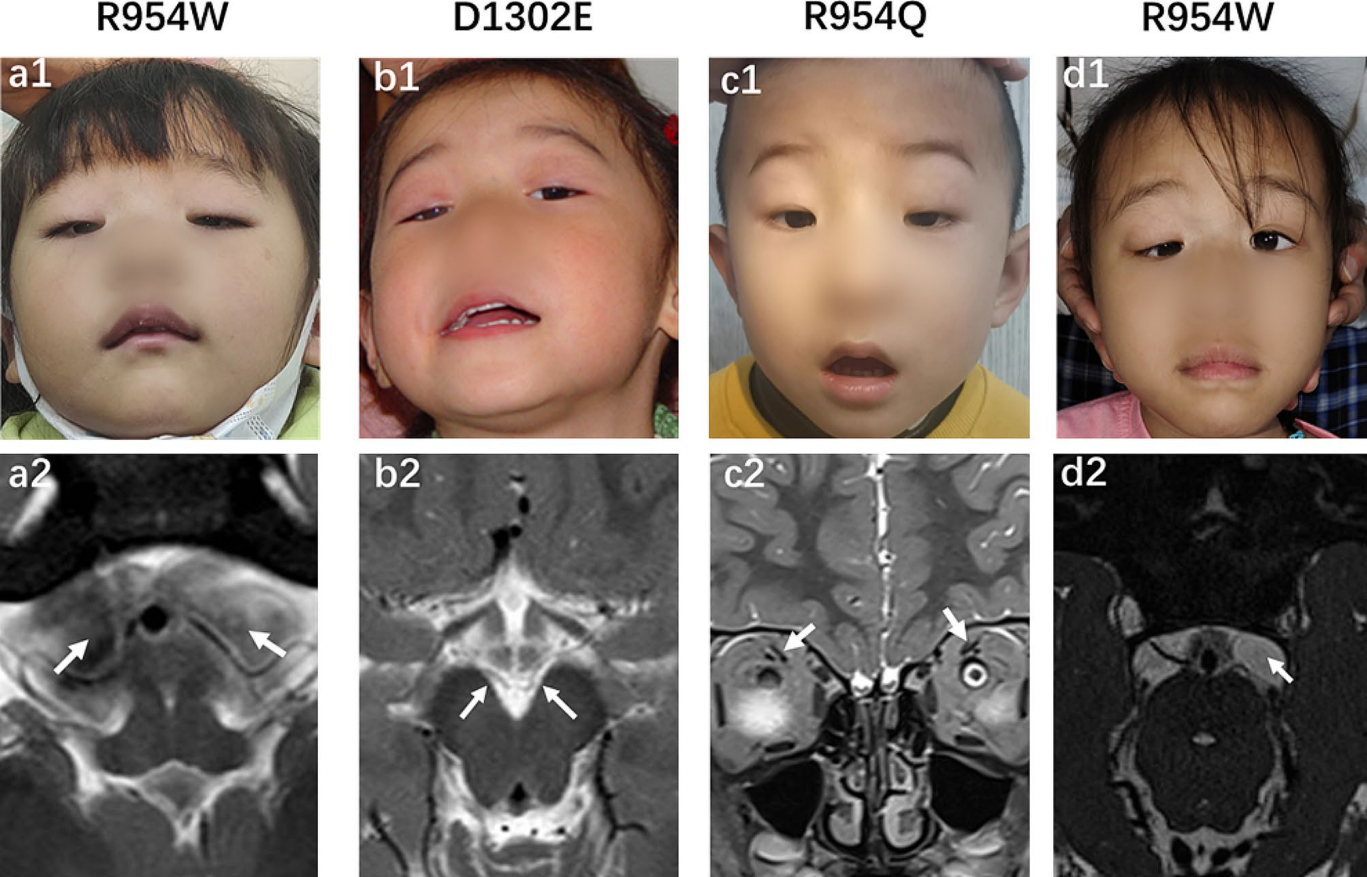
Ocular and MRI findings of patients with CFEOM1 (a1–c2) and CFEOM3 (d1–d2) carrying *KIF21A* variants. a1, a2. Patient S2, harboring the Arg954Trp variant, displayed bilateral ptosis and absence of CN6 on MRI. b1,b2. Patient S4, harboring the Asp1302Glu variant, showed bilateral ptosis and hypoplasia of the bilateral CN3 on MRI. c1,c2. Patient S6, harboring the Arg954Gln variant, exhibited bilateral ptosis and hypoplasia of the bilateral levator palpebrae superioris-superior rectus on MRI. d1, d2. Patient P12-III1, with CFEOM3, harbored the Arg954Trp variant and showed bilateral ptosis and absence of left CN6 on MRI
